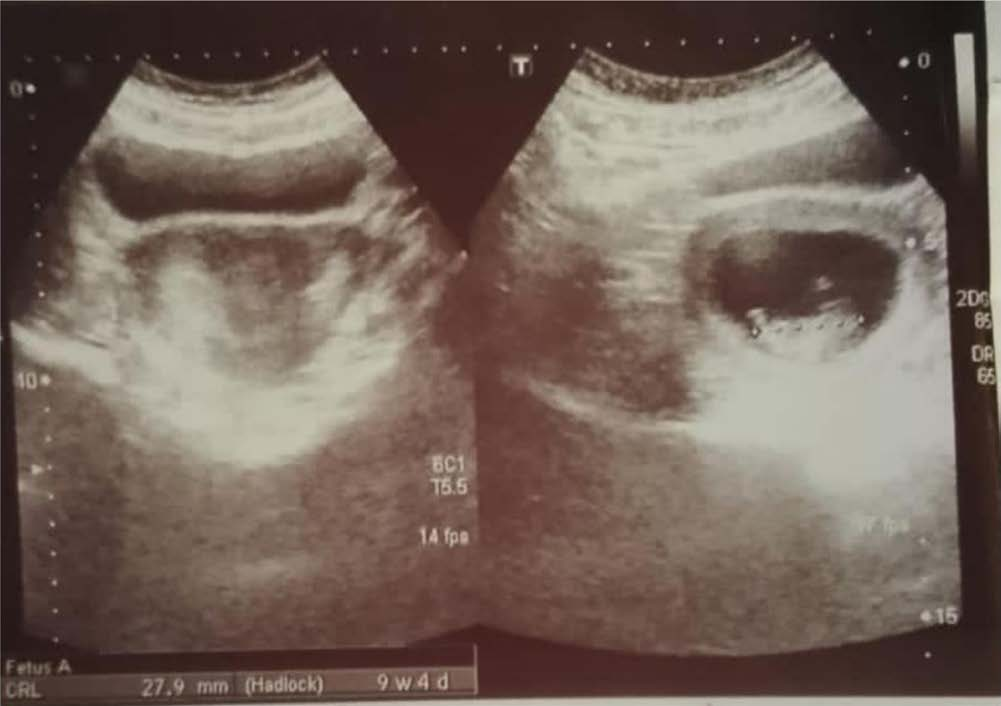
Ultrasound showing a bicornuate uterus.
